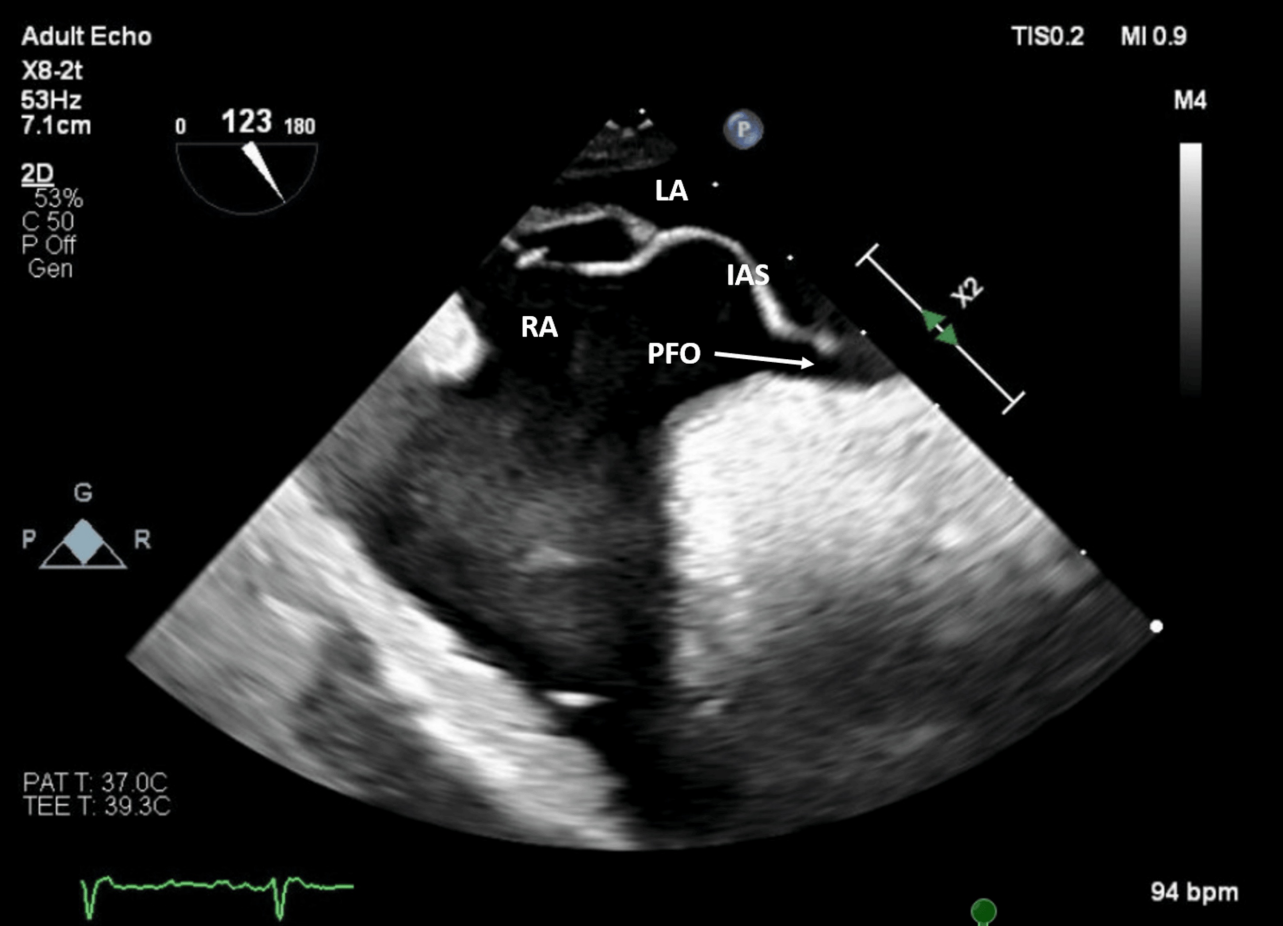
Trans-esophageal echocardiogram (TEE) demonstrating a patent foramen ovale with an aneurysmal interatrial septum RA = Right Atrium; LA = Left Atrium; IAS = Interatrial Septum; PFO = Patent Foramen Ovale
